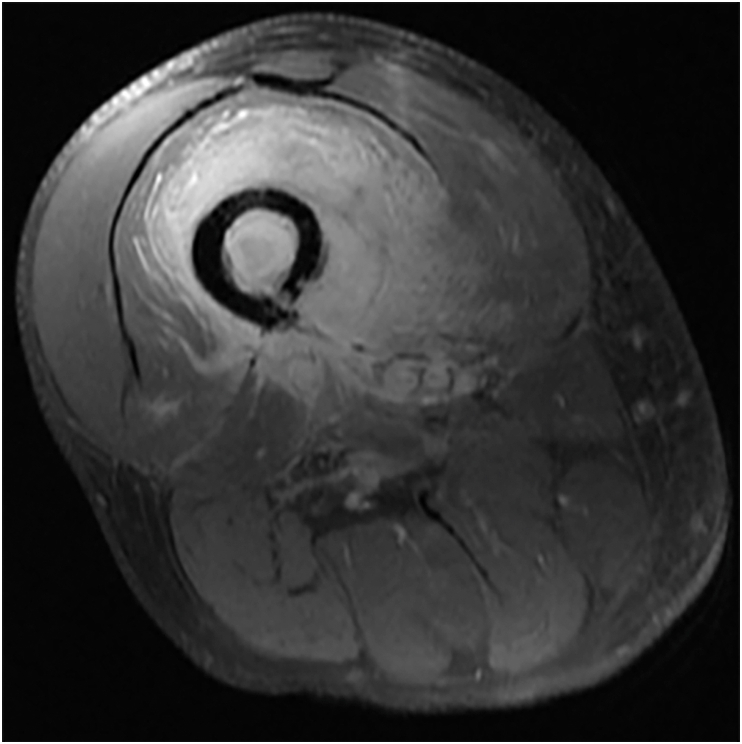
Axial T1 fat suppression post-contrast MRI at time of index presentation demonstrating right femur diaphyseal acute osteomyelitis with extraosseous abscess and pyomyositis.
